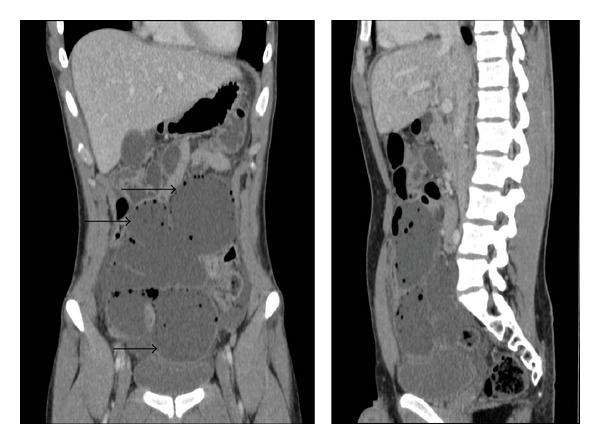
CT scan appearance of the small bowel hamartoma (large diverticula arrows).
